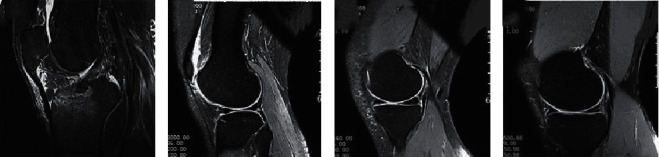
MRI image of meniscus. (a) MRI image of normal meniscus. (b) MRI image of grade I injury in the posterior horn of the medial meniscus (female, 24 years old). (c) MRI image of grade II injury of the posterior horn of the medial meniscus (female, 62 years old). (d) MRI image of grade III injury of the posterior horn of medial meniscus (male, 36 years old).
